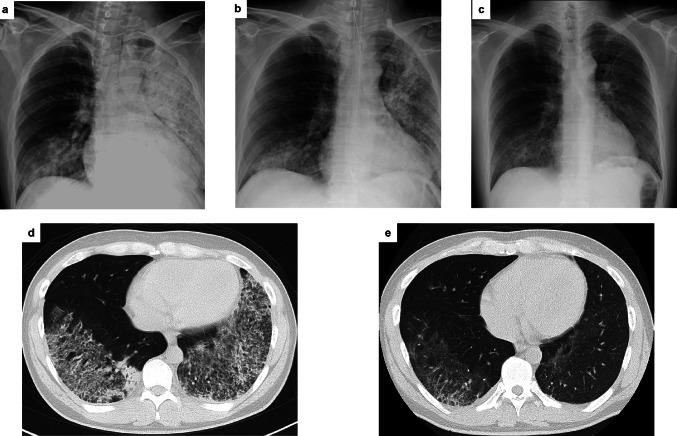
Temporal changes in pulmonary infiltration. Serial chest X-rays on the day of admission (a) and day 7 (b) in the supine position, and on day 12 (c) in the upright position. Serial CT images obtained on the day of admission (d) and day 14 (e)
